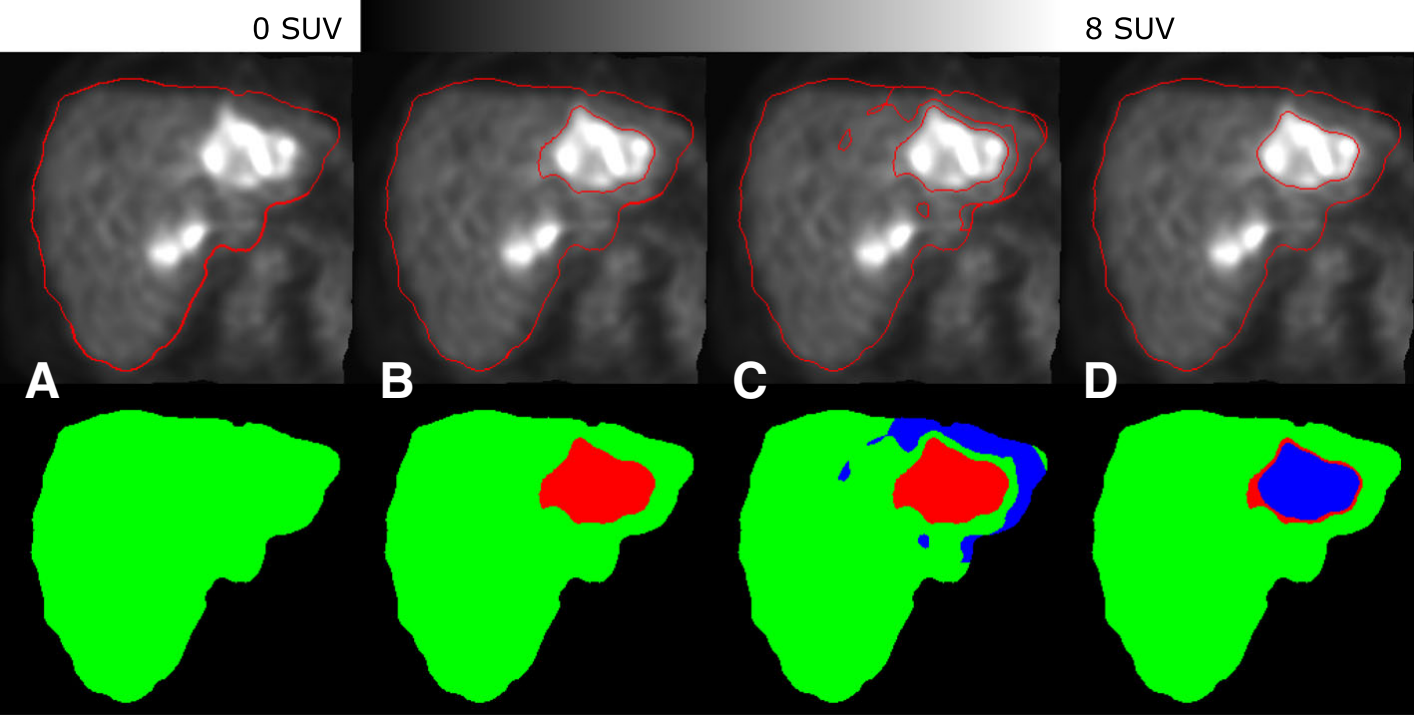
Tumor segmentation steps for one of the tumors (patient ID BI01 from training set in our database) that is visible in the slice by using the method proposed in [78]. For the other tumor(s), the same algorithm was used a initial ^18^F-FDG PET and (liver segmentation: green), b^18^F-FDG initial threshold and finding initial tumor burden (liver segmentation, green ; tumor cores, red), c finding specific background for each tumor (liver segmentation, green; tumor cores, red; background, blue), and d final tumor thresholding (liver segmentation, green; initial tumor volume which is not included in the final tumor segmentation, red; final tumor segmentation, blue)
